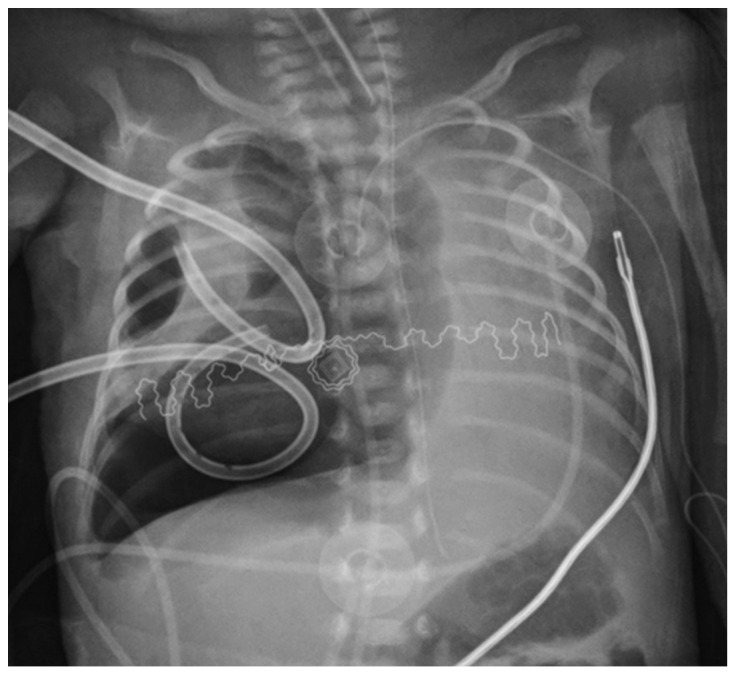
X-ray in 16th day of life. Recurrence of pneumothorax despite two suction chest drains.
